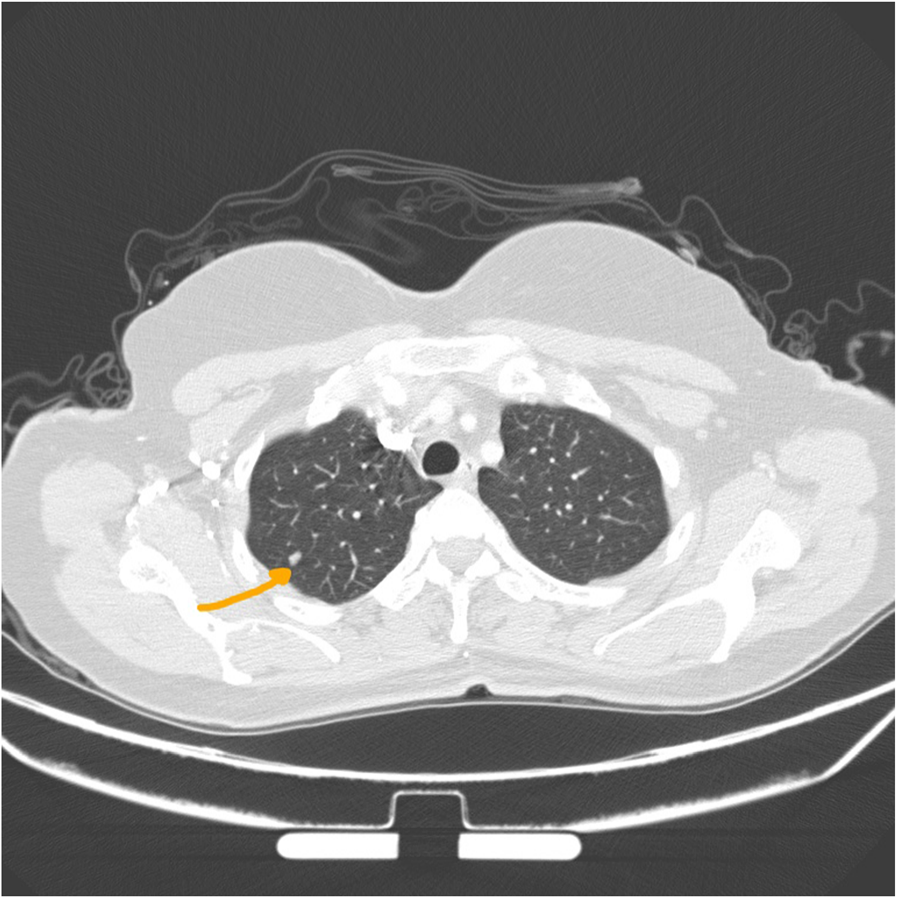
CT scan of the chest showing the small pulmonary nodule in the right upper lobe
